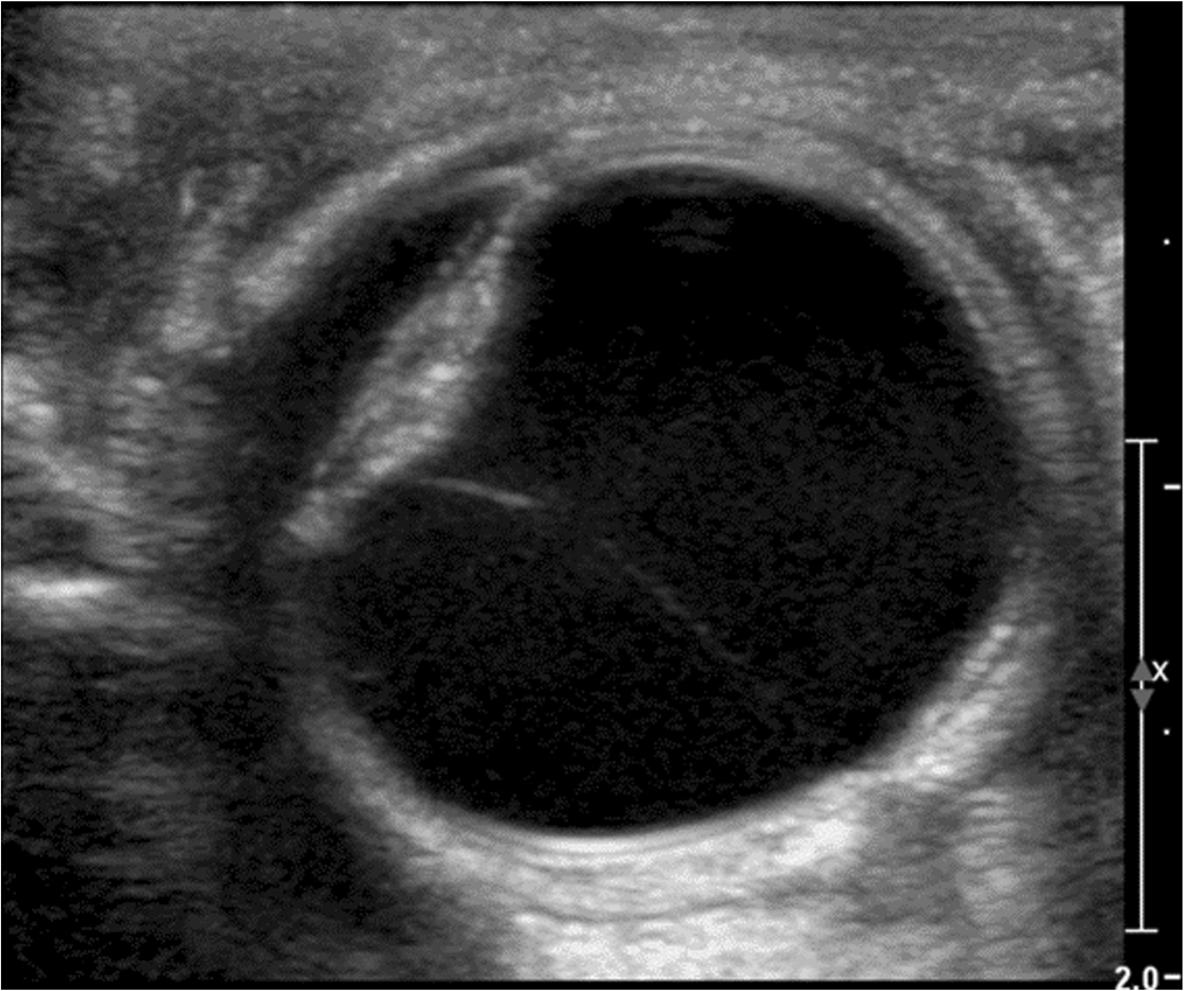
Ocular sonography of Patient 5 at the age of 10 days - Persistent hyperplastic primary vitreous artery. A thin y-shaped membrane extends from the optic disc to the posterior aspect of the opacified lens representing a remnant of the hyaloid vasculary system
